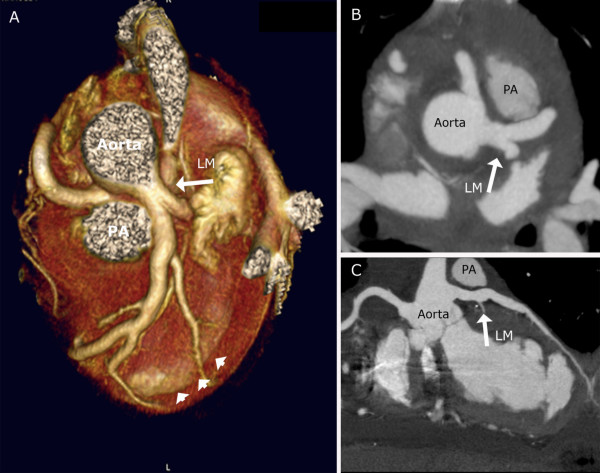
CT-angiography after operation. Coronary CT-angiography one week after aortic reimplantation of the left coronary artery confirms patency. Note the myocardial wall thinning in the apical anterior part of the left ventricle demonstrating the old infarction (arrowheads). Volume-rendered reformation (A), axial (B) and curved multiplanar reconstruction (C).
